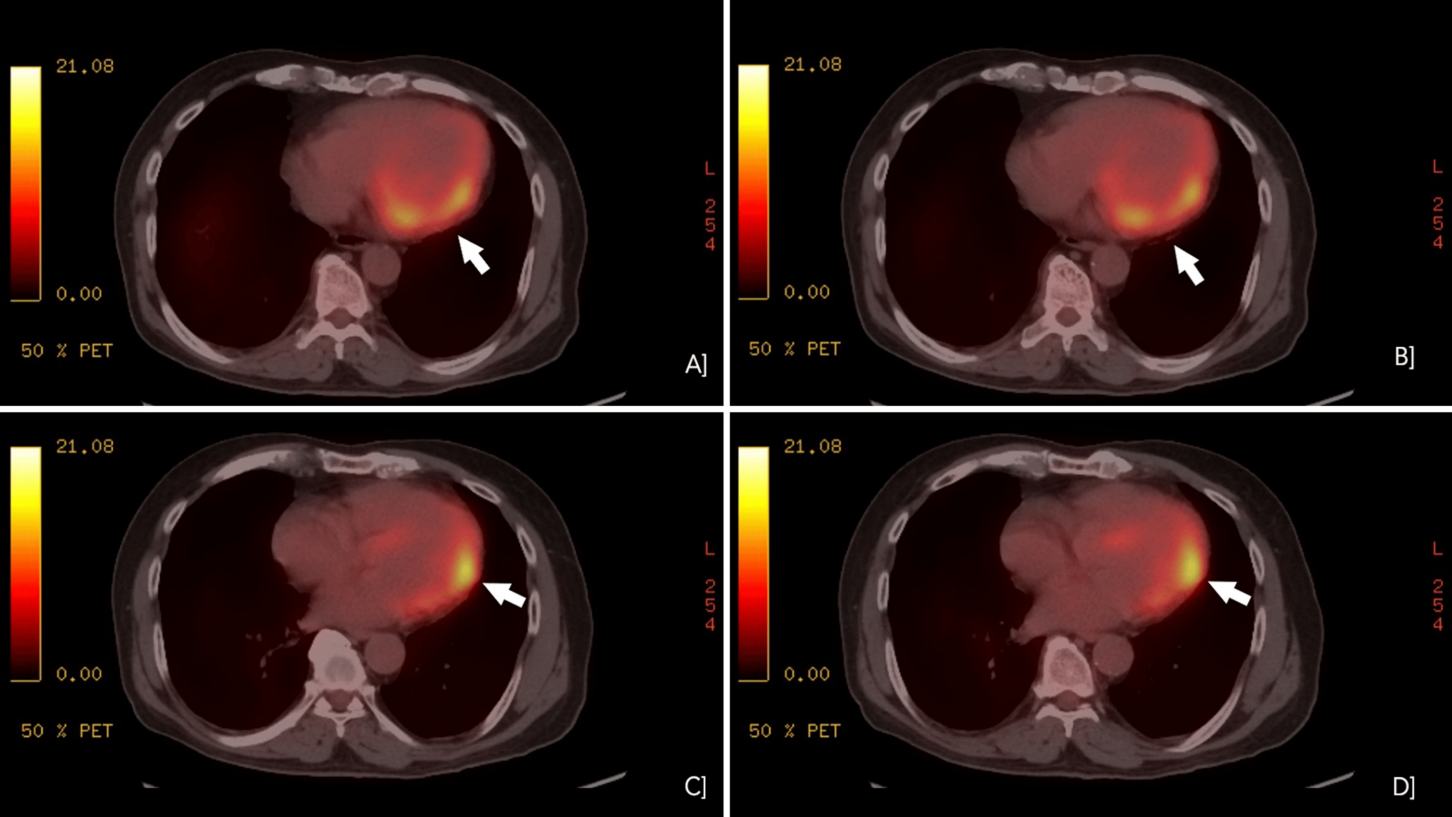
Axial view of different slices of FDG PET-CT showing diffuse hypermetabolism of the anterolateral wall of left ventricle FDG PET-CT: Fluorodeoxyglucose positron emission tomography-computed tomography
